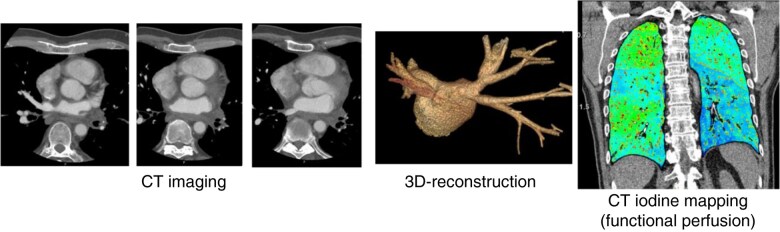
A* and *B*) CT images of a patient with complete occlusion of the left inferior PV after prior PV isolation, *C* and *D*) post-stenting CT with stent open in left inferior PV, *E*) perfusion CT prior to stenting of the left inferior PV demonstrating a large perfusion defect in the left lung (blue colour). CT, computed tomography; PV, pulmonary vein
